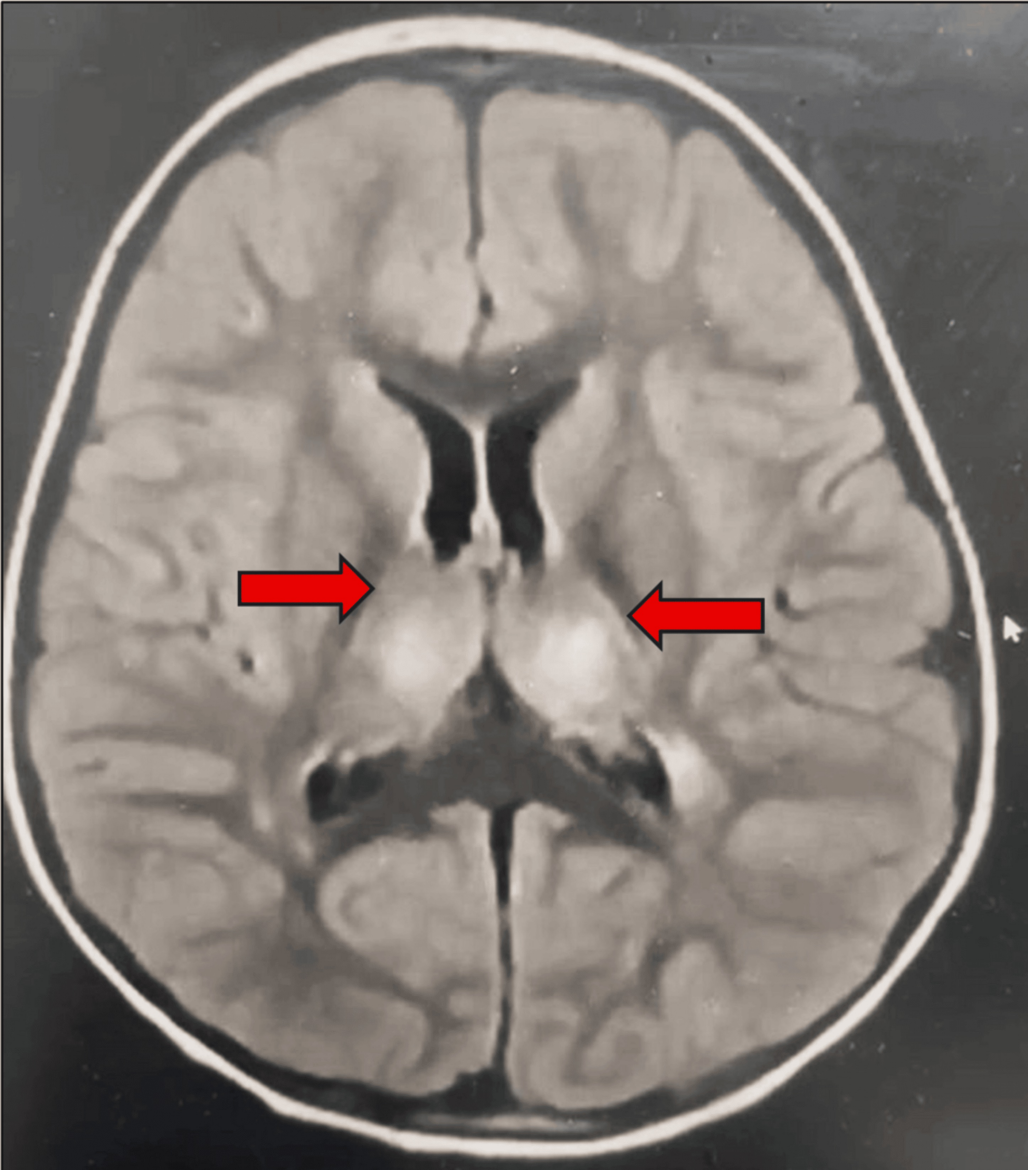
Axial brain MRI (FLAIR sequence) demonstrates bilateral symmetrical thalamic hyperintensities (red arrows). MRI: magnetic resonance imaging; FLAIR: fluid-attenuated inversion recovery
